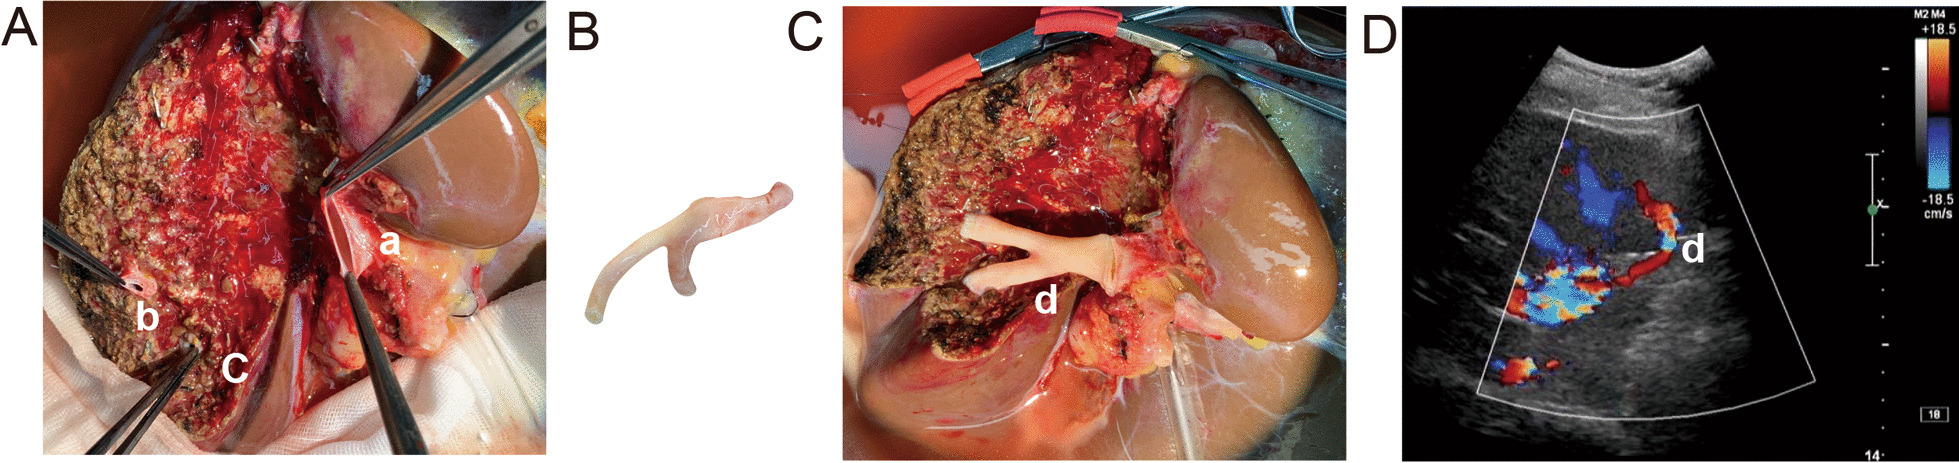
The IV segment portal vein reconstruction. A The extended right grafts. The ‘a stands for the left portal vein, and ‘b’ and ‘c’ mean the IV segment portal vein. B Iliac vein of dornor. C The reconstruction of IV-segment and left portal vein by the iliac vein. ‘d’ stand for the reconstruction vein. D The reconstruction vein by the color doppler ultrasound
